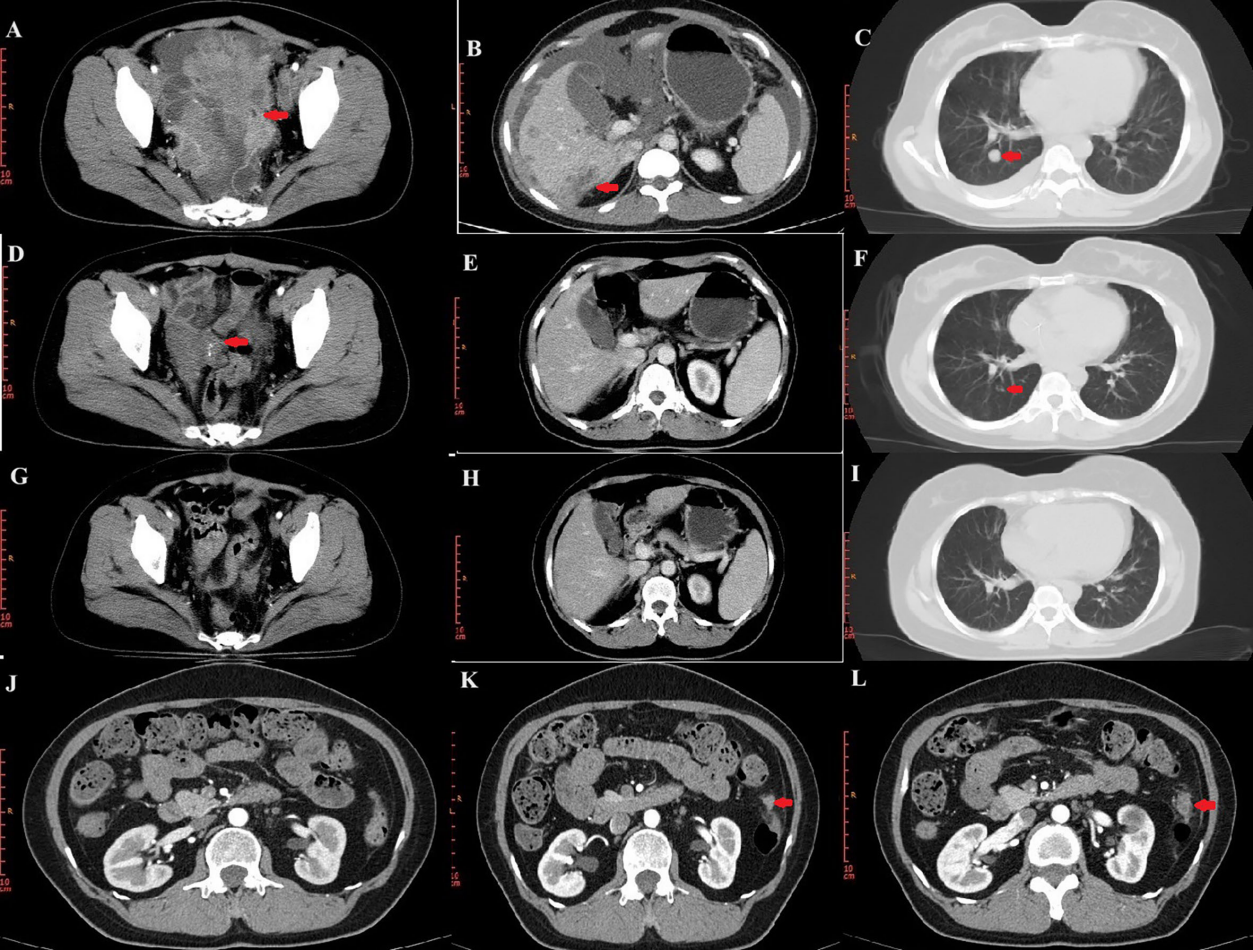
CT images during treatment. (A–C) CT image of the lesion at initial treatment. Pelvic lesions (A). Liver lesions (B). Lung lesions (C). (D–F) CT images after three cycles of chemotherapy. (G–I) CT images at the end of chemotherapy. (J) No tumor recurrence on CT on April 19, 2023. (K, L) Lesions of peritoneum on CT.
